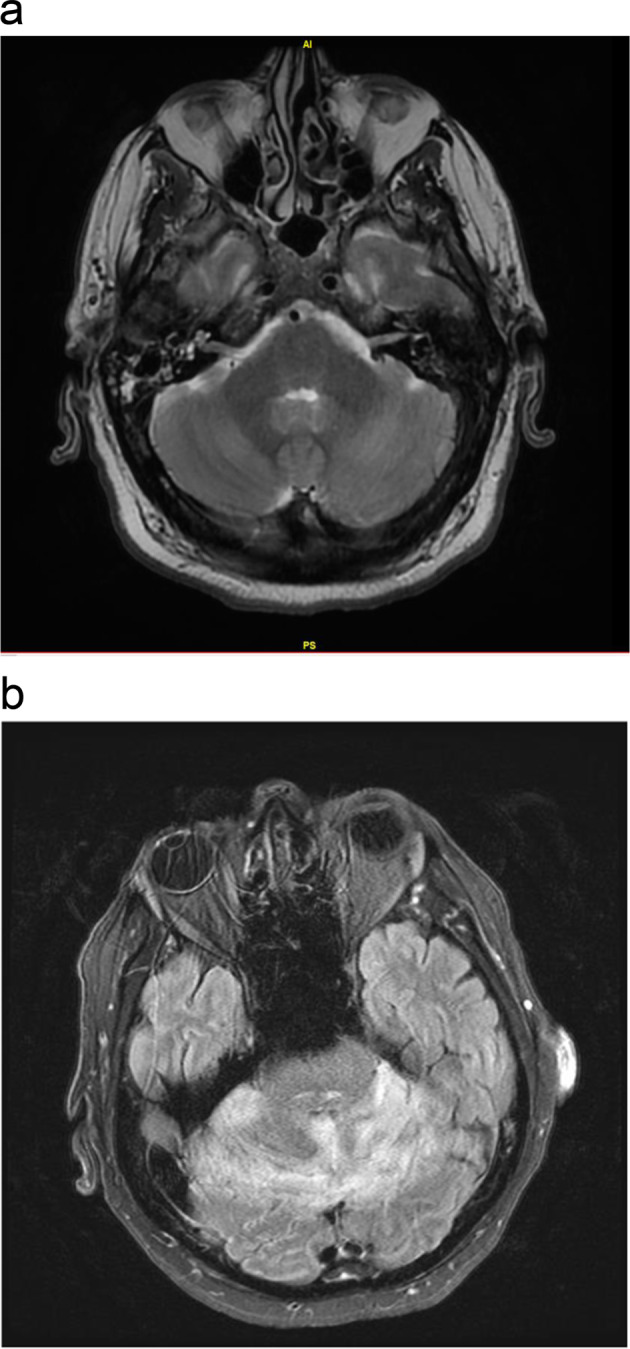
Demonstrates MRI findings of neurologic involvement in Powassan virus encephalitis. a Axial T2 weighted MRI shows T2 signal abnormality in the cerebellum consistent with cerebritis in patient 1. b Axial FLAIR image shows T2 signal abnormality consistent with cerebellar swelling in patient 2.
